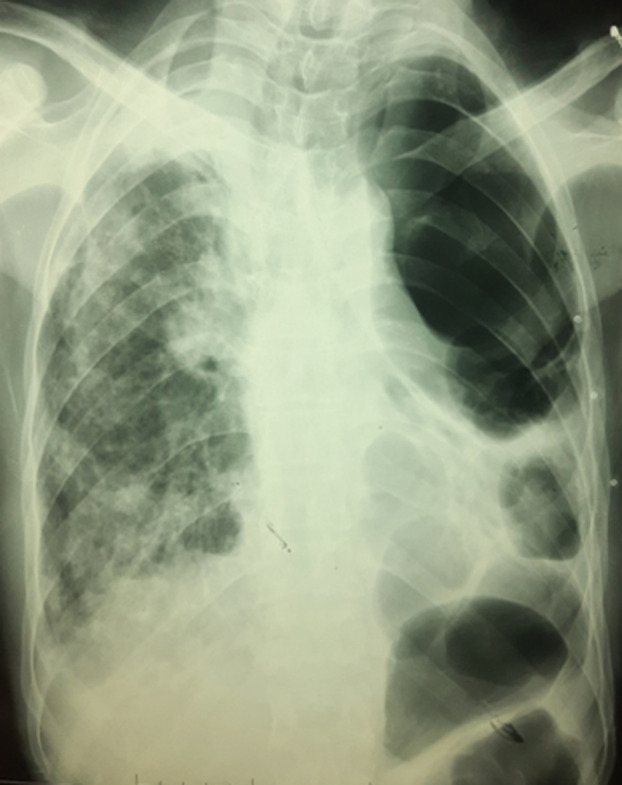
chest X-ray showing diffuse and severe emphysema in the left lung with mediastinal deviation and a reticular shadow in the right lung
